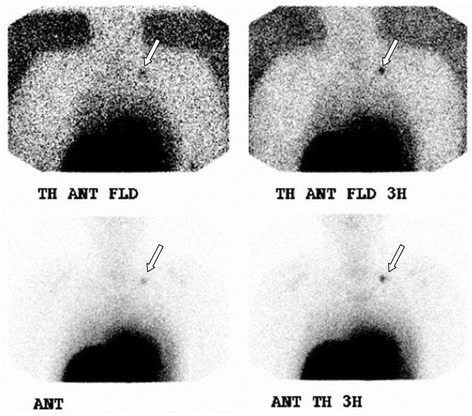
Example of scintigraphic examination (thoracic part) performed 1 h (*left*) and 3 h (*right*) after radioactive tracer injection. A focal area of radioactivity is noticed in the left cervical region
